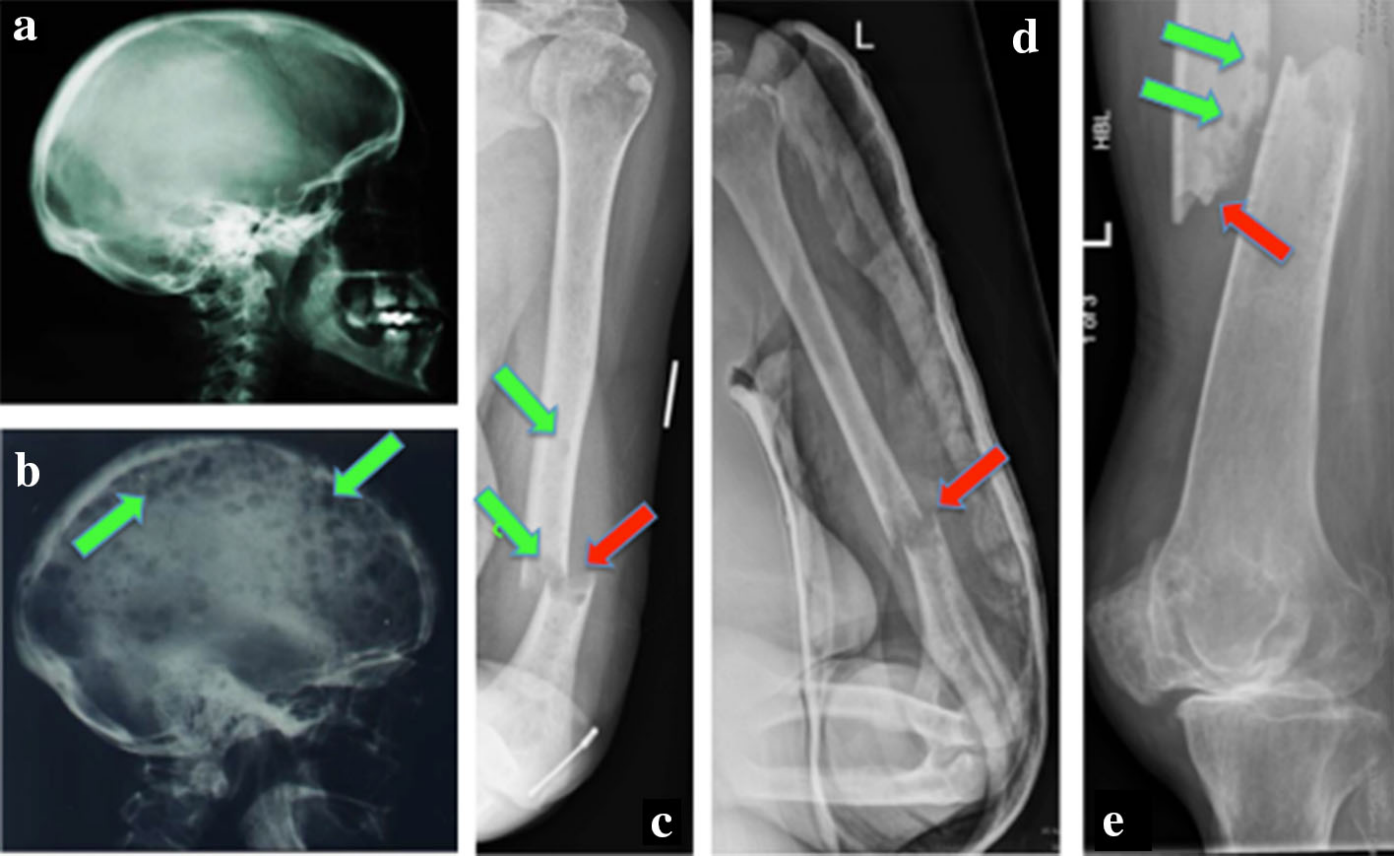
X-ray examples of serious but preventable myeloma-induced osteolytic lesions and pathological fractures potentially preventable if detected earlier. a Normal skull. b Myeloma ‘pepper pot skull’ riddles with lytic lesions. c, d Pathological fractures through lytic lesions in the distal shaft of the left humerus. e Pathological fracture through the proximal shaft of the left femur
